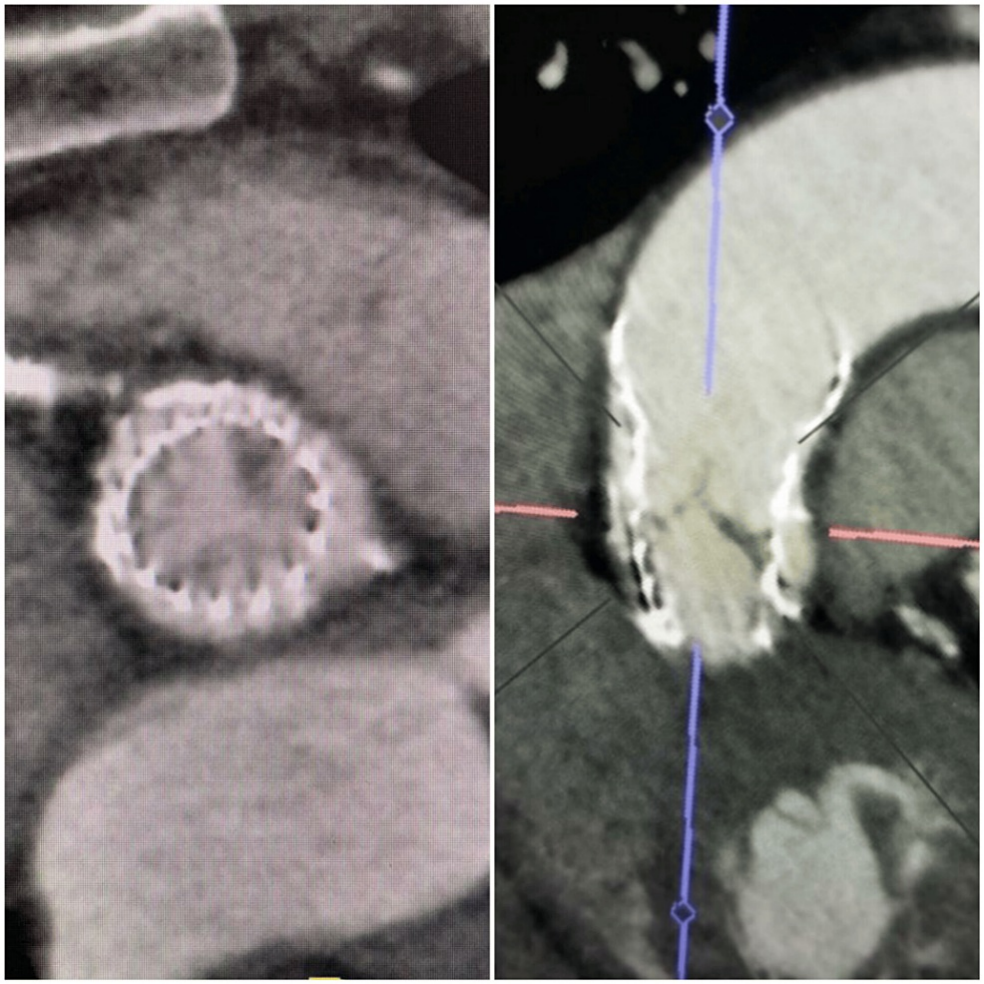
Cardiac CT highlighting HALT in short and long axes CT, computed tomography; HALT, hypoattenuated leaflet thickening
